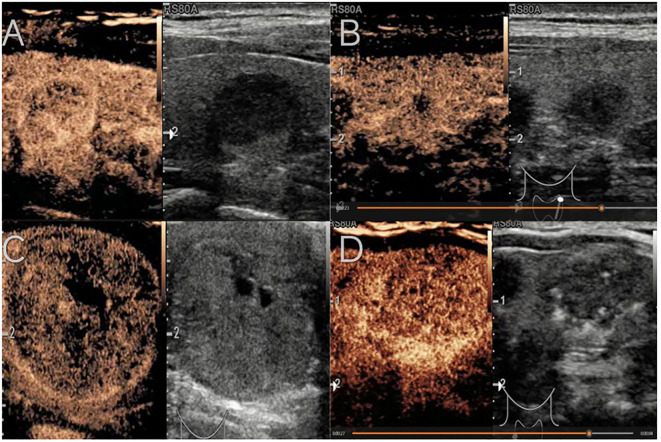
CEUS image (left) and conventional ultrasound image (right). (A) Complete peripheral ring enhancement, benign lesion on FNA. (B) Absent ring enhancement, surgical PTC. (C) Cystic nodule with focal perfusion defect, benign. (D) Solid hypoperfused nodule, PTC.
